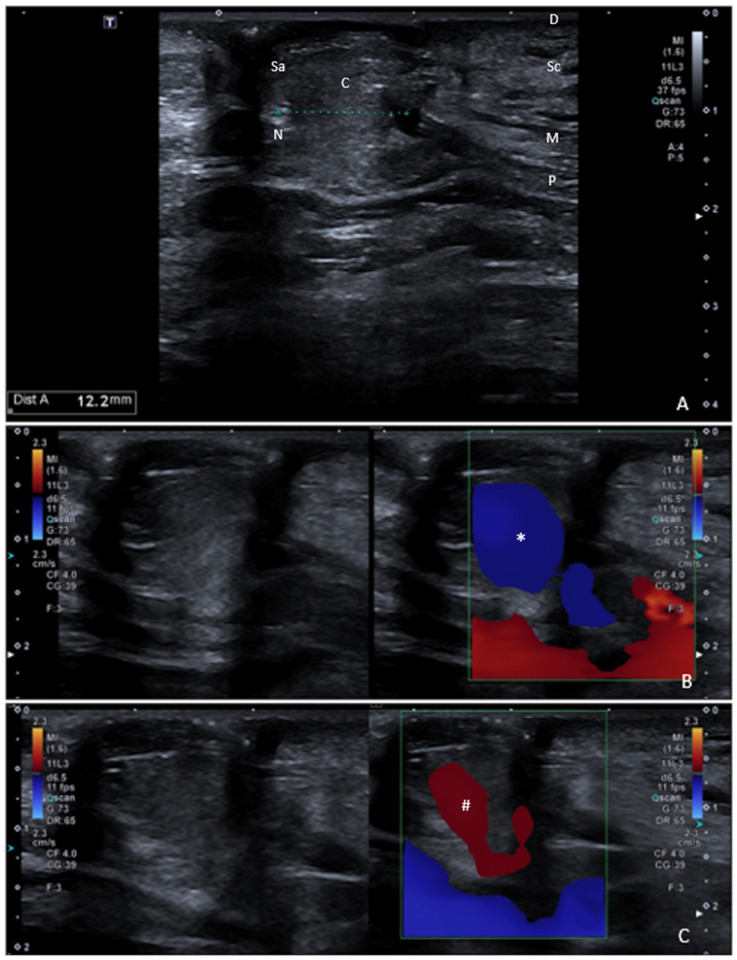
Linear probe B-mode ultrasound imaging showed umbilical hernia (A), with three key elements: sac (Sa), neck (N), and content (C) [1]. In our patient, the content consisted of omental fat; however, hernias can contain other tissues too, such as small and/or large bowel loops [2]. Anterior abdominal wall superficial layer consists of very thin epidermis, thicker dermis (D), and underlying subcutis (Su). Myofascial layer is the middle one, and consists of muscles and their fascial envelopes (M). Transversalis fascia, preperitoneal fat, and the parietal peritoneum forms the deep layer (P) [3,4,5]. Probe pressure was applied and the hernia partially retracted into the abdominal cavity, generating a blue color on Doppler ultrasound (*, (B)). Probe pressure was released, and a red Doppler signal was visible over the hernia, which was moving towards the probe (#, (C)). This sequence demonstrated the movement of the hernia, indicating at least a partially reducible hernia under probe pressure (Video S1, Figure S1). In color Doppler mode, when the object is moving away from the transmitter it encounters less oscillations per unit time than its stationary equivalent, so the frequency of the reflected wave is apparently reduced; instead, when the object is moving towards the ultrasound wave, the frequency of the reflected wave is increased. Usually, the reduction and increase in the reflected waves frequency are, respectively, depicted with blue and red colors in the image [6,7,8,9]. As the patient stands up still, the probe is gently placed over the hernia site without compression and the Dual Image (B-mode/color Doppler) is activated, setting the Pulse Repetition Frequency to about 15 cm/s. During the probe pressure, the hernia sac motion through the hernial gap appears as a blue color Doppler signal moving away from the probe, indicating at least a partially reducible hernia. When the probe pressure is released, the hernia sac motion toward the probe causes the appearance of a red Doppler signal over the hernia. Instead, as the patient lies in a supine position, the Cocco sign consists of the detection of the movement toward the probe of the abdominal wall hernia sac during the Valsalva maneuver, and of its retraction upon the cessation of the Valsalva maneuver [10]. Therefore, there are some differences between probe-induced Cocco sign and the Cocco sign. First, in the former, the patient stands up, whereas in the Cocco sign the patient lies in a supine position. Second, in the former, the patient does not need to do anything, whereas the Valsalva maneuver is required in the Cocco sign. Third, in the former, the pressure applied to the probe determines the movement of the hernia if it is at least partially reducible. Fourth, the cessation of the probe pressure allows the hernia to move forward again. It should be noted that the Valsalva maneuver can be related to some limitations in the Cocco sign technique, such as in patients who are unable to perform the Valsalva maneuver due to respiratory or other medical conditions. Therefore, the probe-induced Cocco sign can overcome these limitations, and, to the best of our knowledge, this is the first report about probe-induced Cocco sign.
